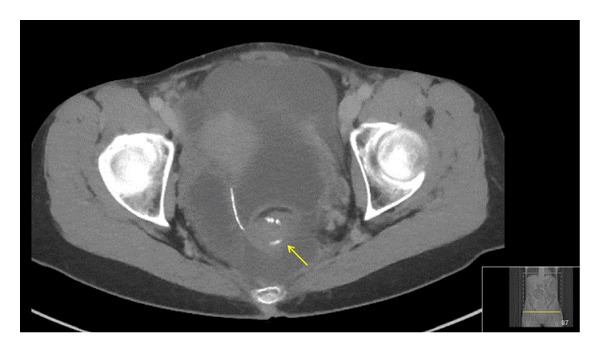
CT-scan showing volvulus of the pelvic pouch apparent by twisting of the posterior staple line 270 degrees just above the level of the ileoanal anastomosis (arrow).
